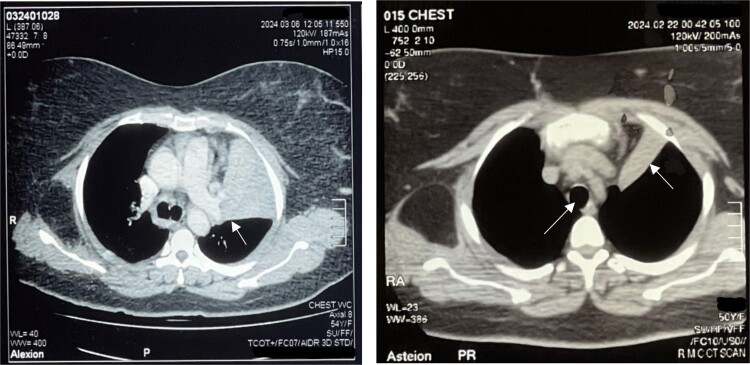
Left: Right main bronchus shown by black arrowhead and inflamed lung parenchyma shown by arrowhead. Right: Trachea and collapsed lung on CT scan (white arrowhead).
